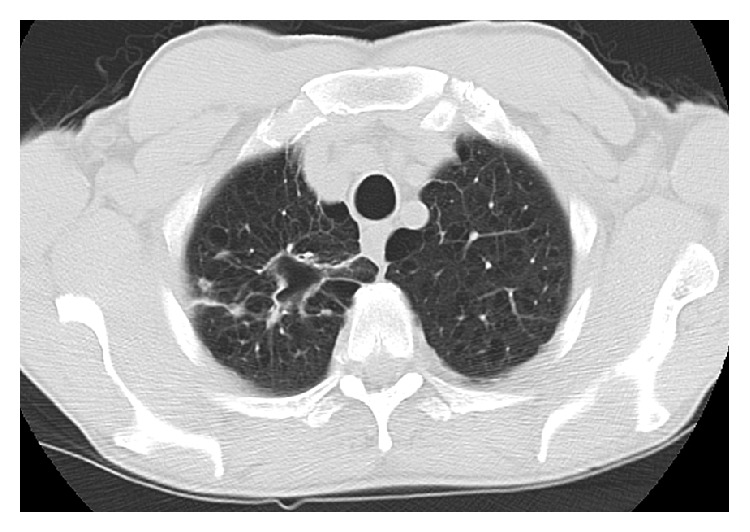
A follow-up CT chest after 6 weeks of antimycobacterial therapy revealed an interval resolution of the right pleura-based mass, and the right upper lobe cavity became thin-walled and much less prominent without new pulmonary lesions or lymphadenopathy.
